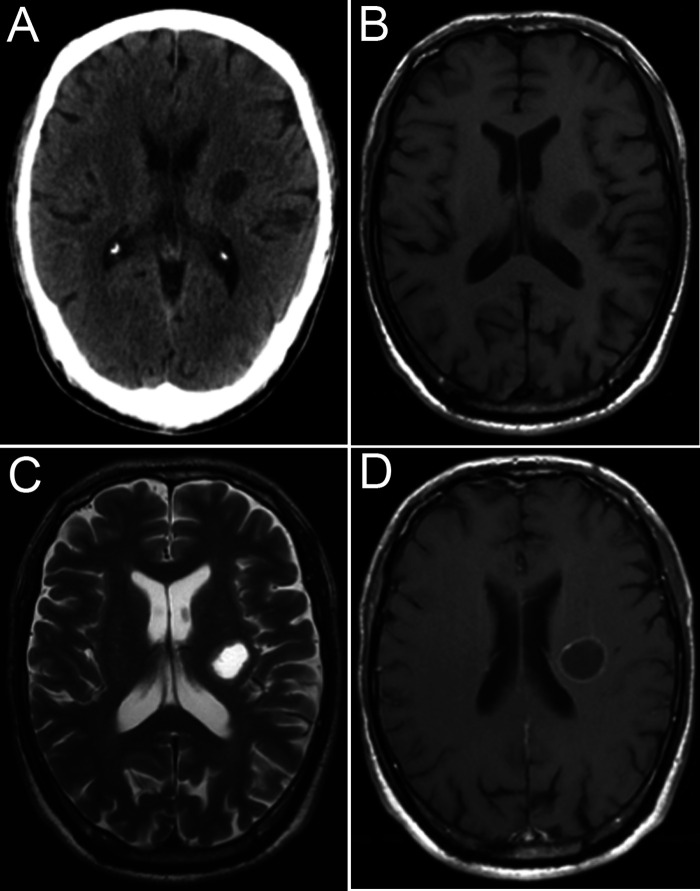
Image at admission. CT image (A), MR T1 image (B), MR T2 image (C), and MR T1-weighted image (D).
